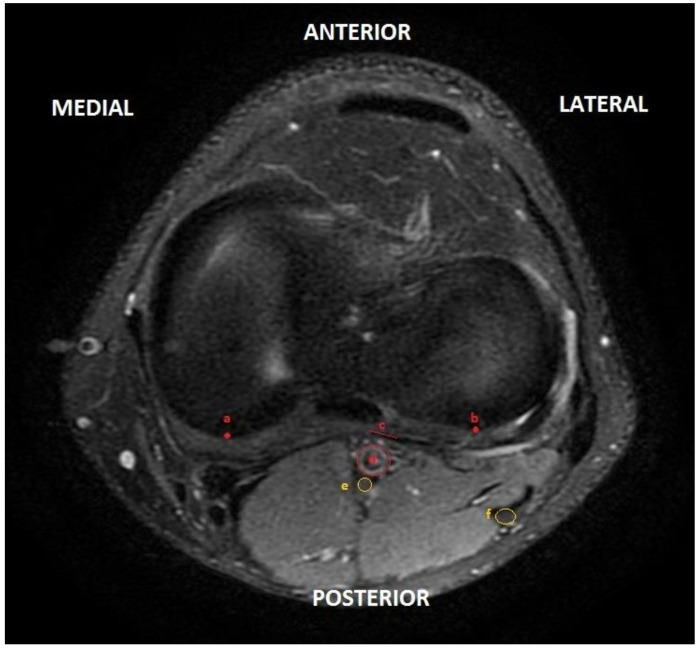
Axial section of the left knee in magnetic resonance imaging, with marks of the main elements: (a) center of the internal tibial plateau at the posterior level; (b) center of the external tibial plateau at the posterior level; (c) posterior capsule next to the popliteal artery; (d) popliteal artery; (e) internal popliteal sciatic nerve (tibial); (f) external popliteal sciatic nerve (common peroneal).
